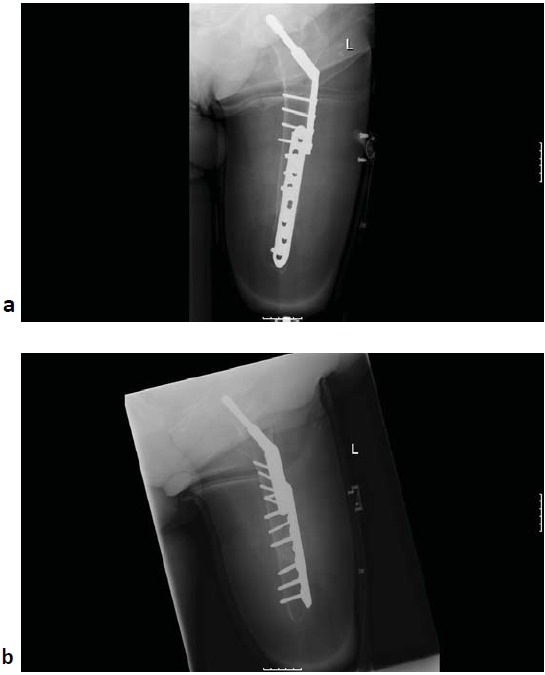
Antero-posterior (a) and lateral (b) radiograph following open reduction and internal fixation of the distal femoral fracture.
